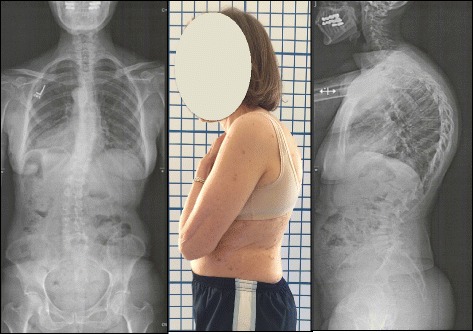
X-ray and clinical picture at evaluation of patient (March 2014): Thoracic kyphosis measured at 86° and lumbar lordosis at 70°
